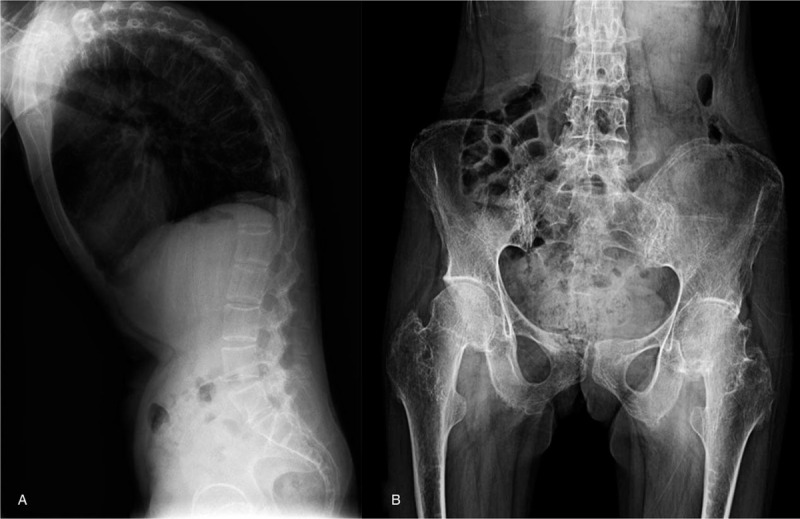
Case 2. (A) x-Ray showing thoracic kyphosis, squaring of the vertebral bodies, sclerosis of some vertebral body edges, blurred facet joints with partly disappearing joint spaces, and calcification of the anterior longitudinal ligament. (B) x-Ray showing blurred surfaces of the sacroiliac joints bilaterally.
